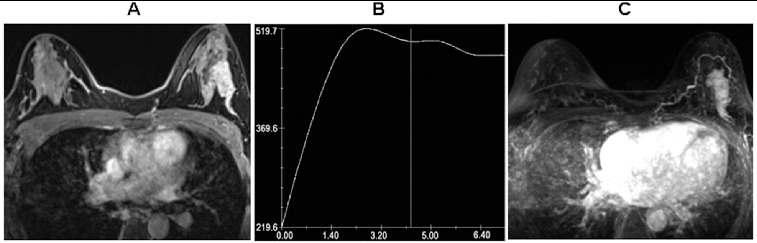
Hyperplasia with intraductal papilloma from a 27-year-old woman. (A) Axial fat-suppressed contrast-enhanced image showing a 2.2 × 2.9 cm irregular mass with heterogeneous enhancement in the upper-outer quadrant of the left breast. (B) Time–signal intensity curve of the mass shows a type II (plateau) time curve. The *x*-axis shows the dynamic imaging beginning time in seconds, and the *y*-axis shows the intensity in arbitrary units. The mass was classified as a BI-RADS category IV lesion. (C) Maximum intensity projection reconstruction obtained from 3D T1-weighted subtraction image showing an adjacent vessel in contact with the edge of the mass.
